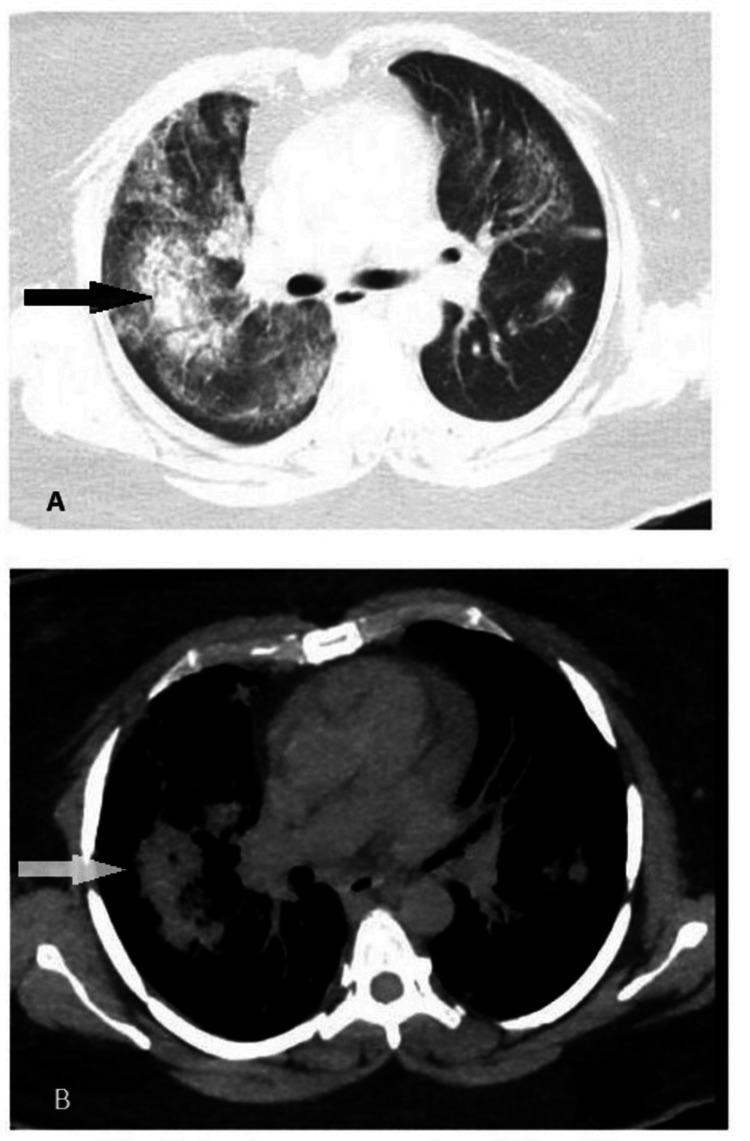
Different images (A and B) of the CT scan of the chest. White and black arrows point toward the areas of diffuse alveolar hemorrhage, which are ground glass opacities.
